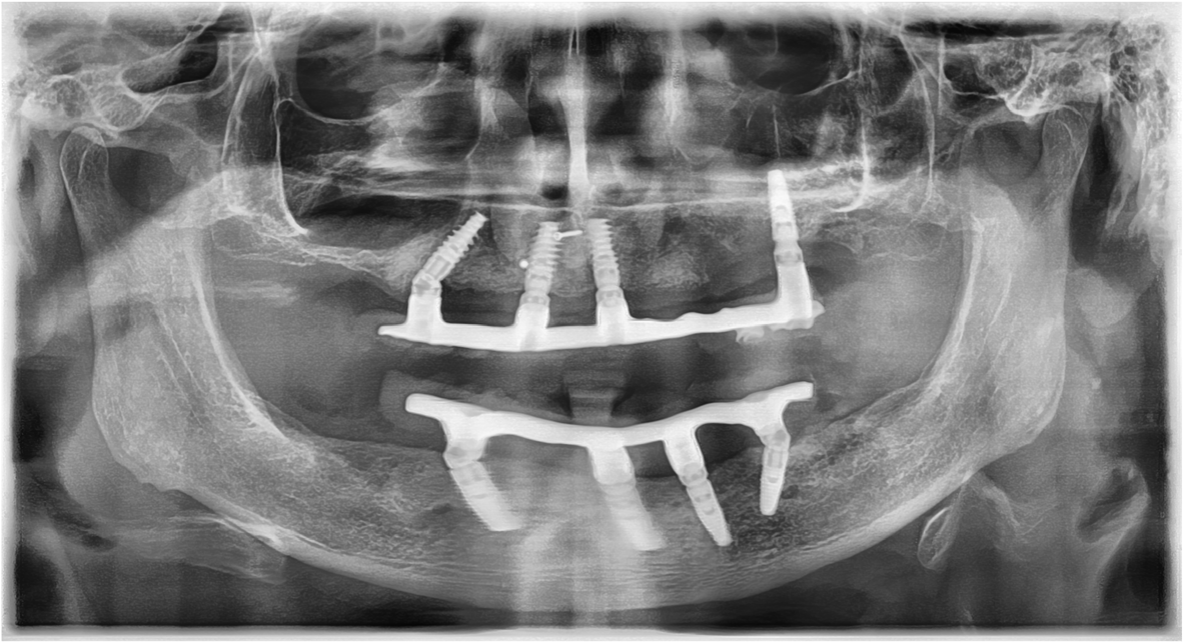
This X-ray records the integration of the redesigned restoration of the case in Figs. 2 and 3. The loosened implant was explanted
